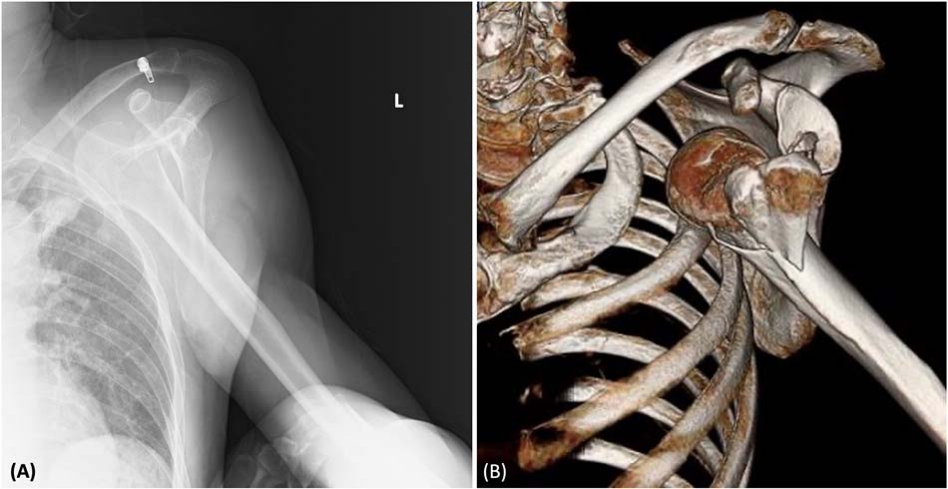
(A) Radiography showing the dislocation of the left shoulder joint. (B) 3D-CT showing a humeral fracture.
